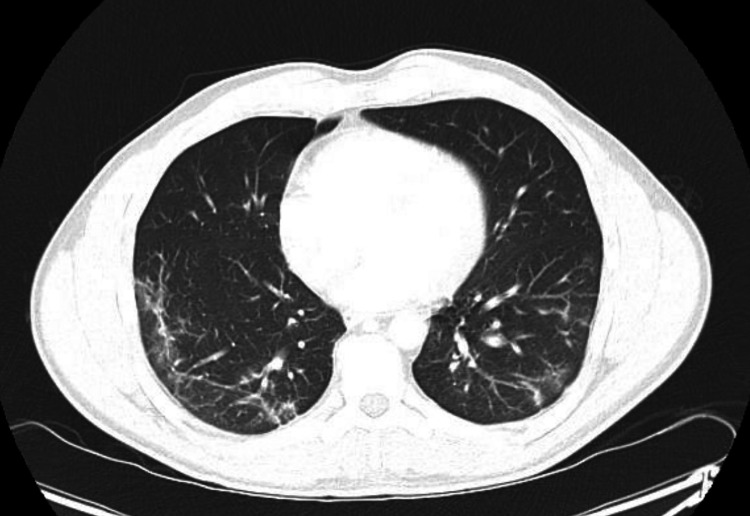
CTA of the chest. Computed tomography angiography (CTA) scan of the chest showing multifocal patchy infiltrates with slight mosaic pattern but no noted cavitary lesion.
